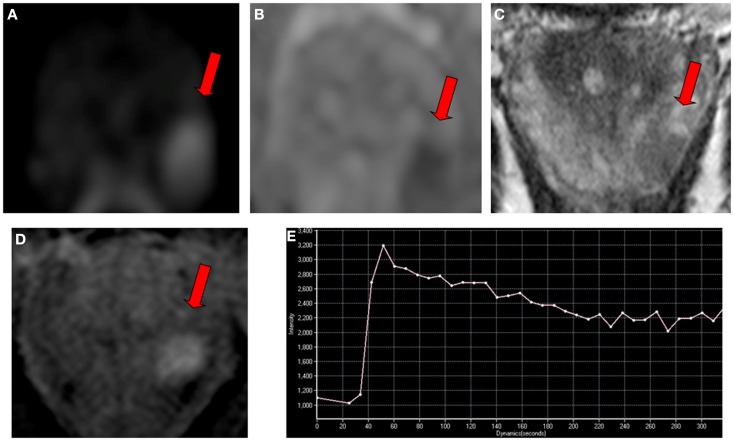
Multi-parametric magnetic resonance imaging in a 65-year-old man referred for early detection of prostate cancer with an elevated serum PSA level (7 ng/ml) and a normal digital rectal examination. Magnetic resonance imaging consisted of conventional T2-weighted, diffusion-weighted, and dynamic contrast-enhanced sequences performed on a 3 T unit without endorectal coil. (A) An ovoidal hyperintense lesion (arrow) was observed on axial diffusion-weighted imaging at *b*-value 1000 s/mm^2^ in left apical peripheral zone. (B) The lesion (arrow) corresponded to hypointense lesion on apparent diffusion coefficient map. (C) The lesion (arrow) also corresponded to axial T2-weighted image with low signal intensity, and to (D) focal enhanced lesion on axial dynamic contrast-enhanced imaging with a type 3 enhancement curve (washout) (E). The final Prostate Imaging Reporting and Data System score for this lesion was four. The presence of a clinically significant cancer was confirmed with a targeted biopsy, showing an 8-mm Gleason score 4 + 3 adenocarcinoma.
